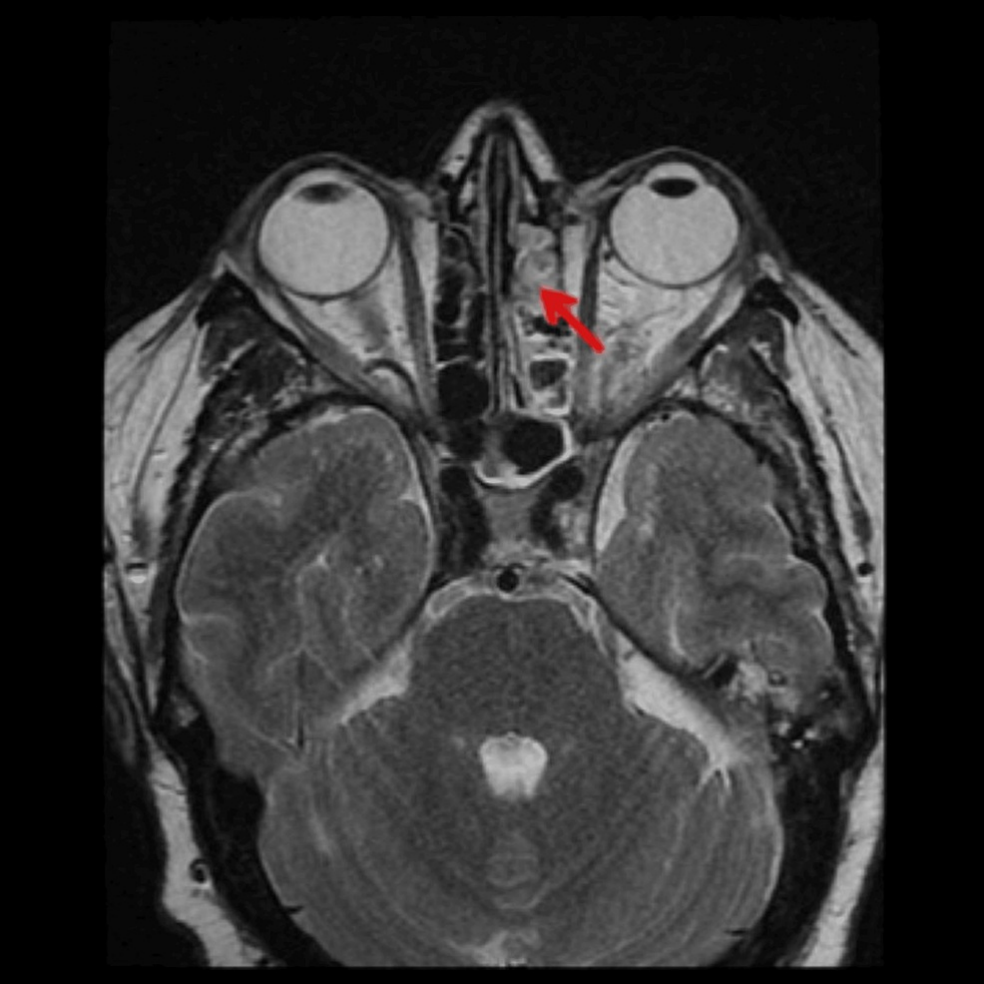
MRI (T1 image) showing mucosal thickening in the left maxillary sinus (red arrow)
